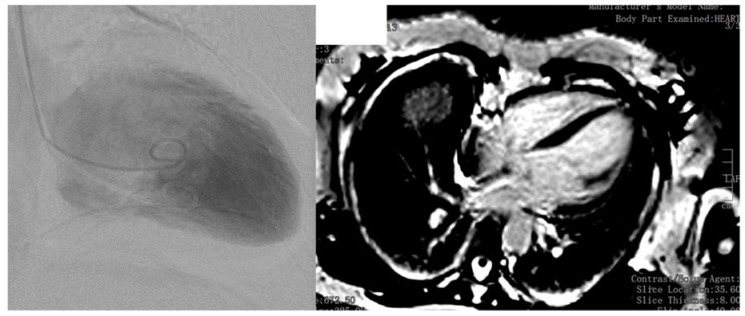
Ventriculography showing ballooning of the apical region of the left ventricle (left panel). Cardiac magnetic resonance imaging, 2 years after the initial event, showing no signs of late gadolinium enhancement (right panel).
